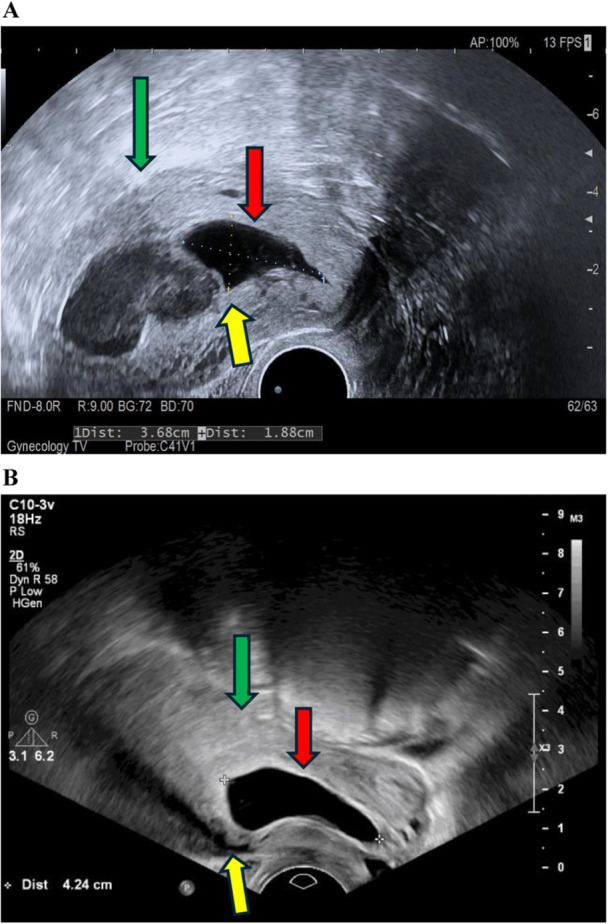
Transvaginal ultrasound images showing type 1 (A) and type 2 CSP (B). The gestational sac (red arrow) is implanted at the site of the previous cesarean scar (yellow arrow), located below and separate from the uterine cavity (green arrow).
